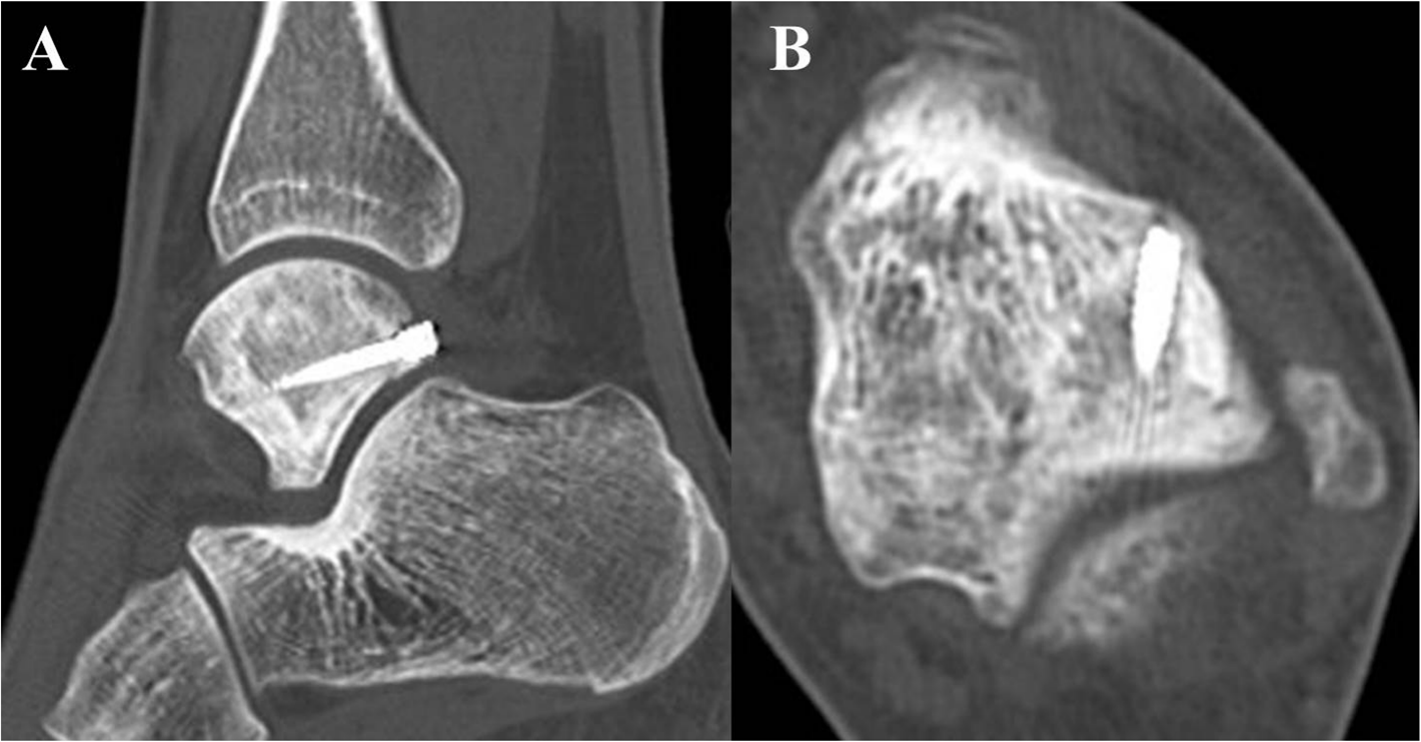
Postoperative non-contrast CT scan. a Sagittal and b axial views at 10 weeks postoperatively showing consolidation of the stress fracture
